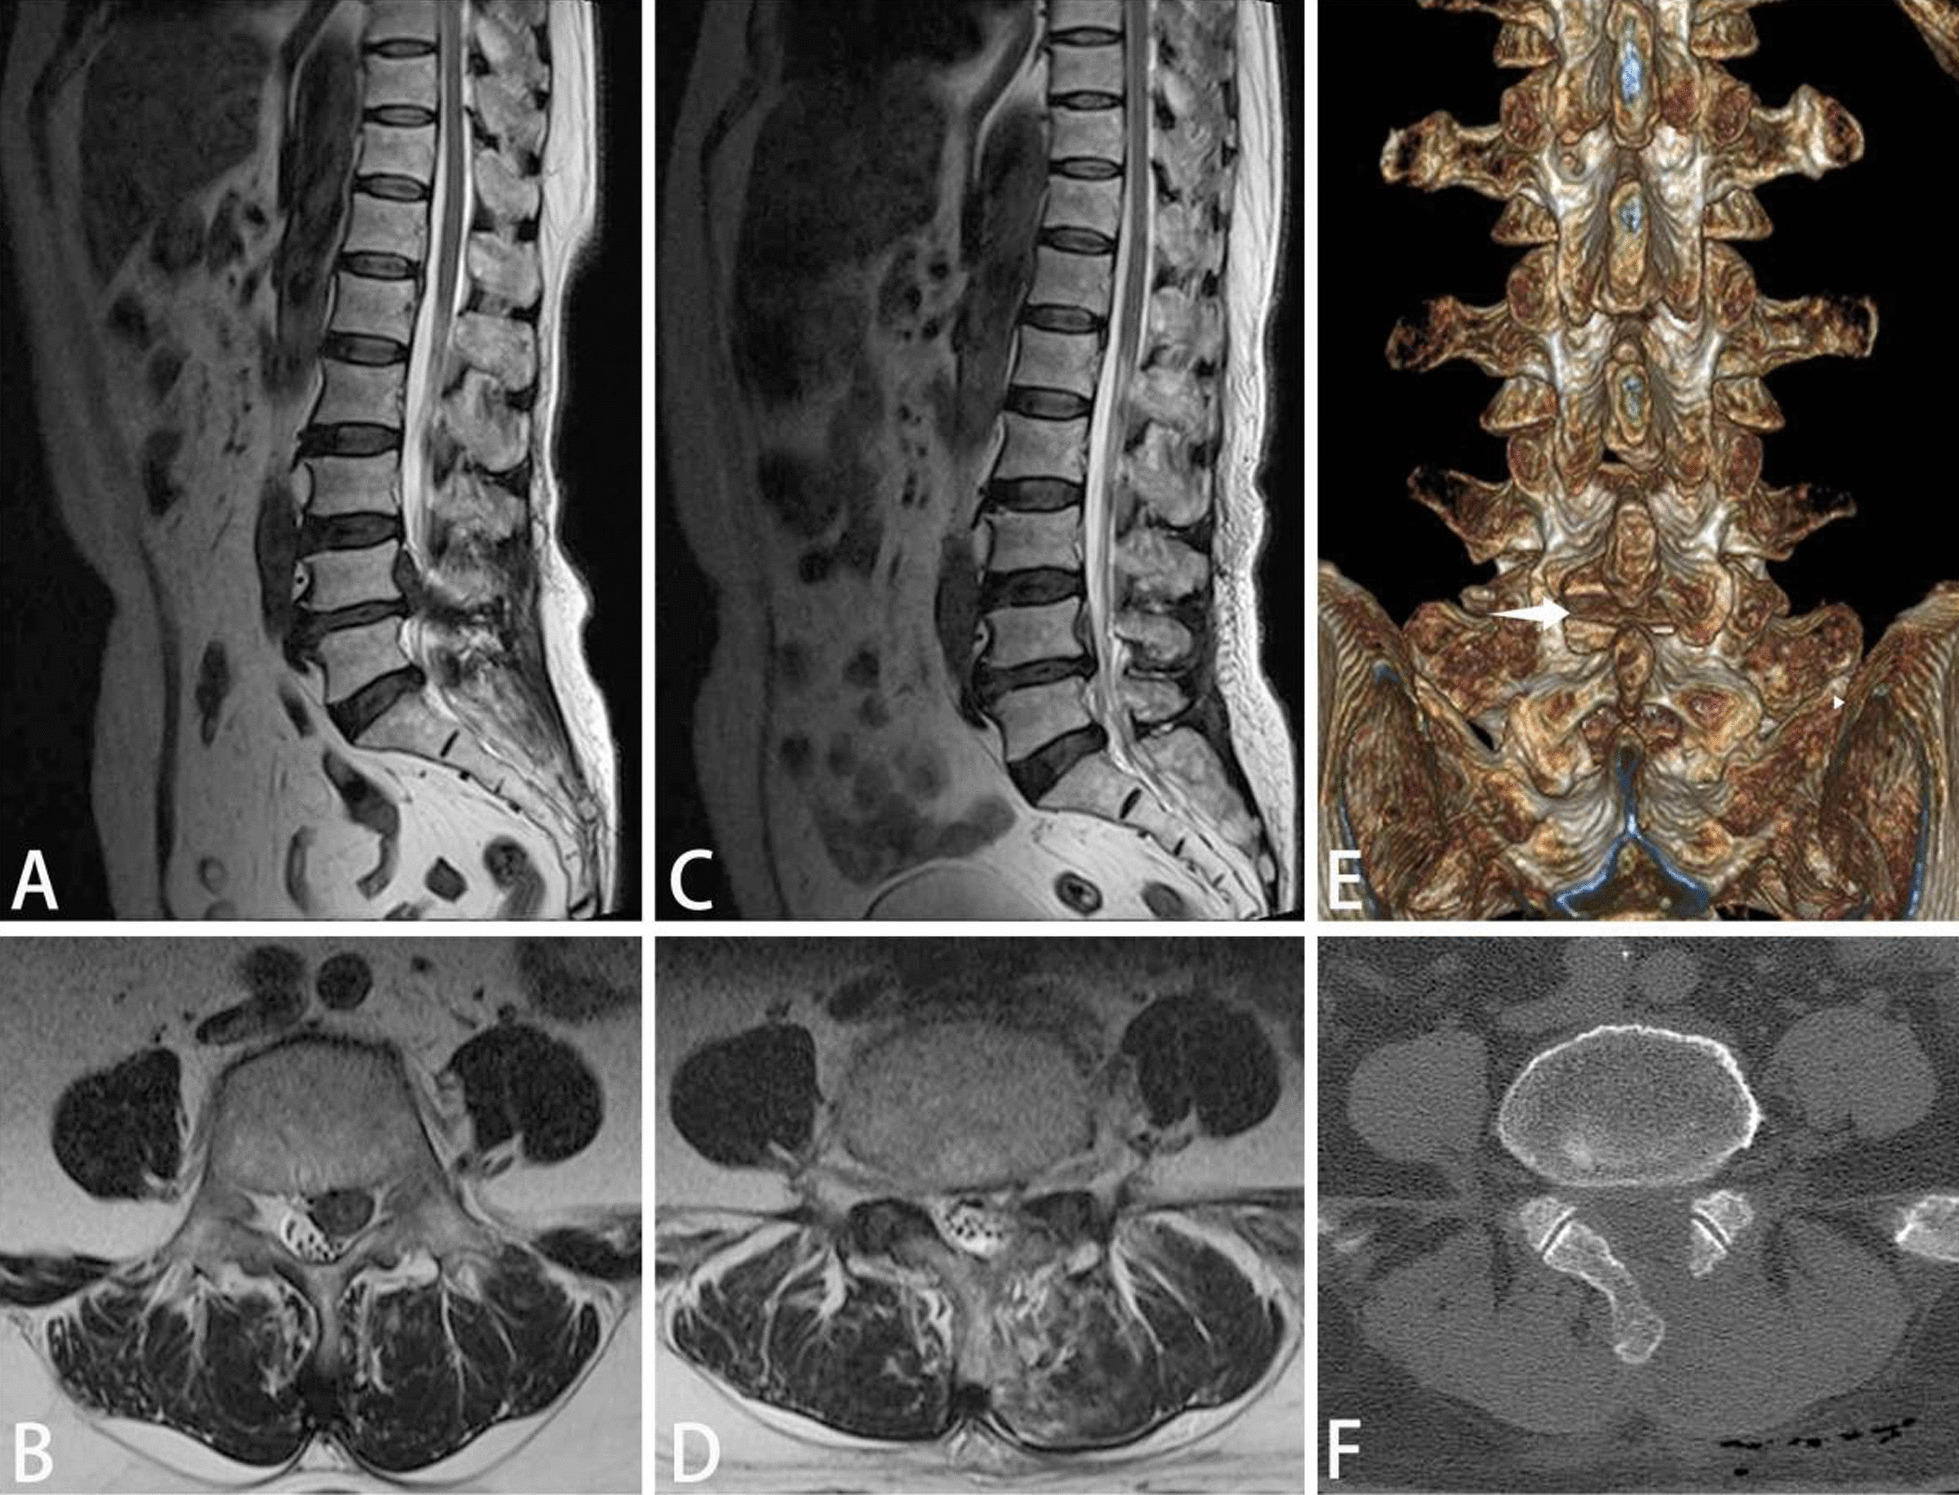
A 65-year-old female patient was diagnosed as type B1 according to the modified classification. The nucleus pulposus was removed through the posterior approach. A–B: The migrated nucleus pulposus was seen on preoperative MRI. C–D: Postoperative MRI showed that the migrated nucleus pulposus was completely removed. E: The bony tunnel of the lamina was seen in the postoperative CT three-dimensional image. F: postoperative vertebral CT showed partial bone defect in the left lamina
